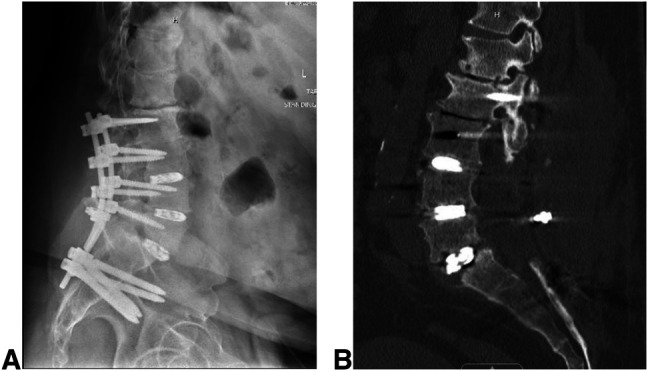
A, Lateral radiograph of the lumbar spine demonstrating a broken rod of the previous construct. B, Radiograph showing the sagittal cut of a CT scan of the lumbar spine demonstrating pseudarthrosis of L5-S1 with subsidence of a transforaminal lumbar interbody fusion cage.
